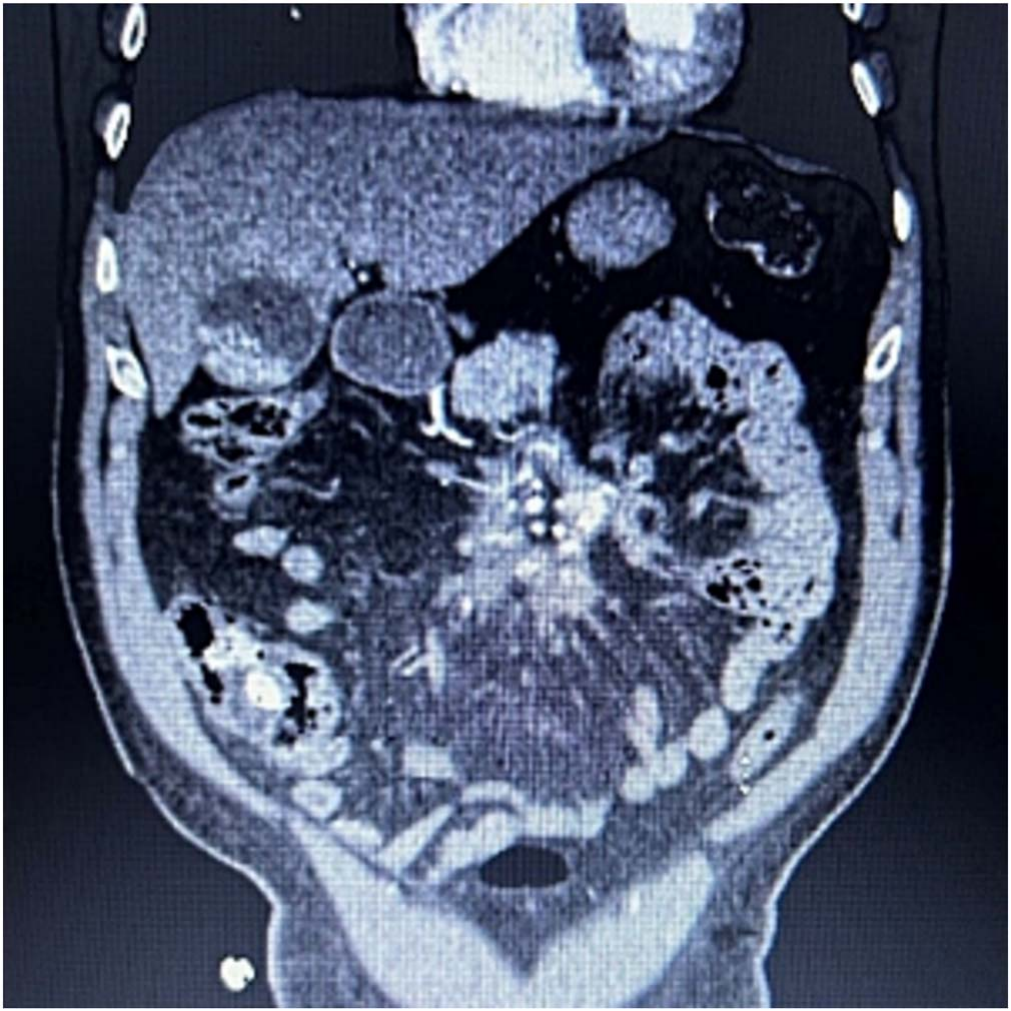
CTA abdomen/pelvis showing central calcified mesenteric mass, Case 2.
